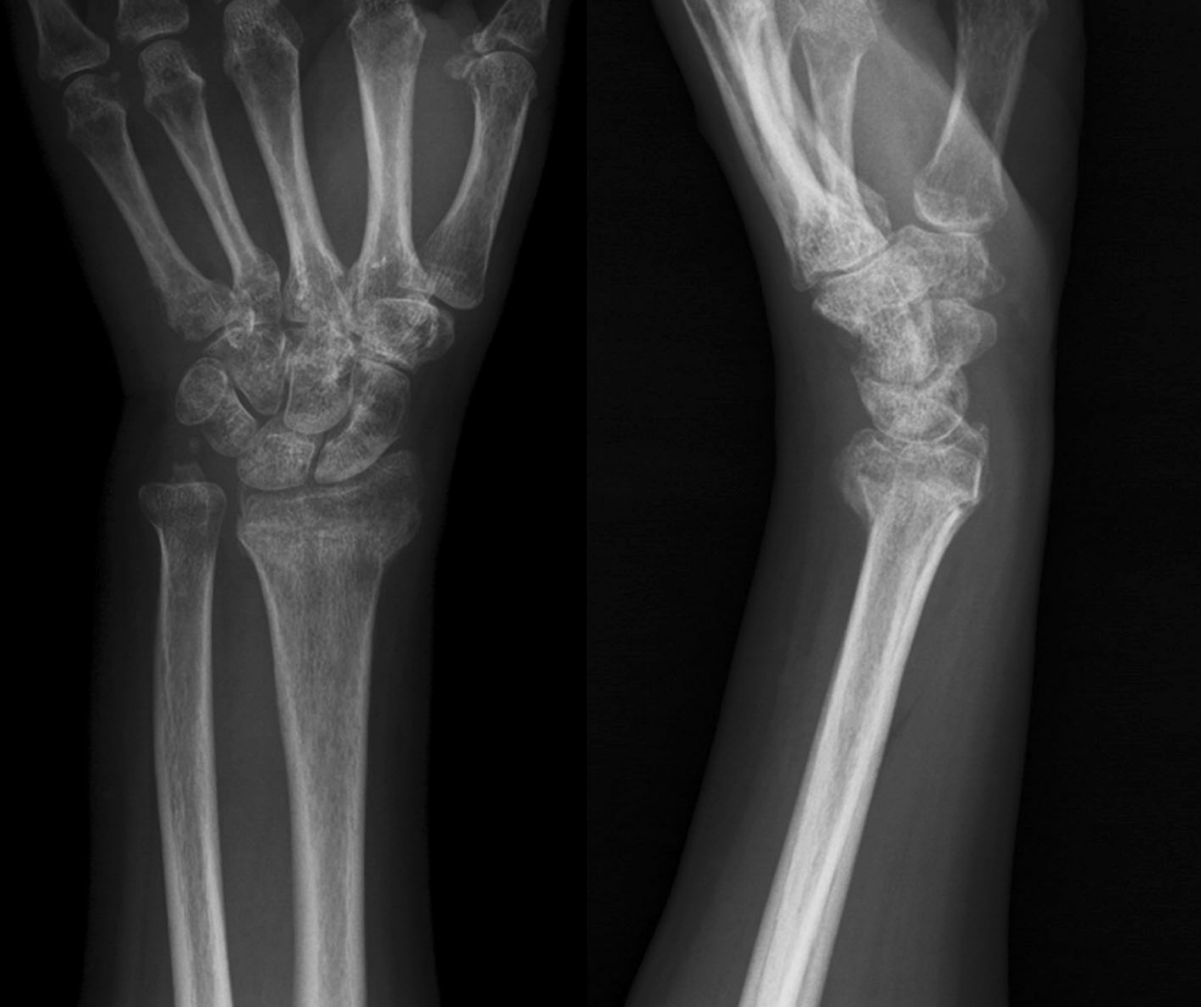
A 69-year-old female patient. Radiographs of left wrist at 6 weeks after injury show fracture of the distal radius with malalignment
